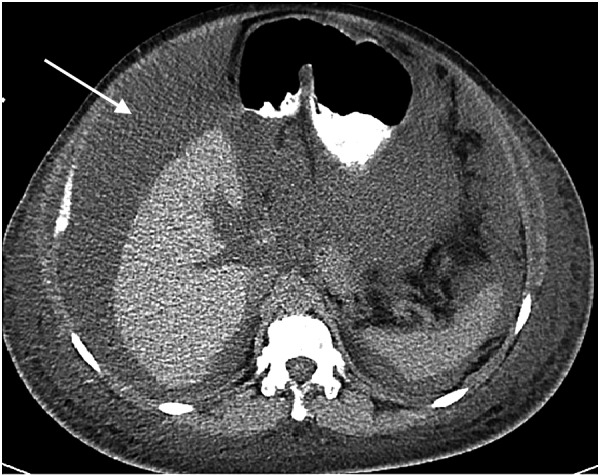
Computed tomographic (CT) scan of the abdomen showing marked amount of abdominal ascites (arrow) with fluid surrounding the spleen and the liver.
